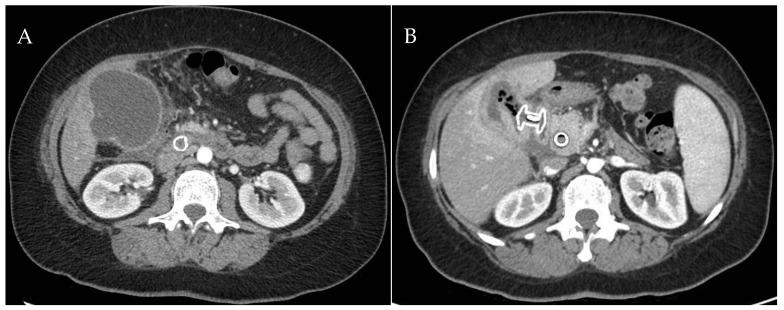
(A) CT scan image of acute cholecystitis after biliary SEMS placement in a jaundiced patient with pancreatic head cancer. (B) Drained gallbladder after LAMS placement. CT: computed tomography; SEMS: self-expanding metal stent; LAMS: lumen-apposing metal stent.
